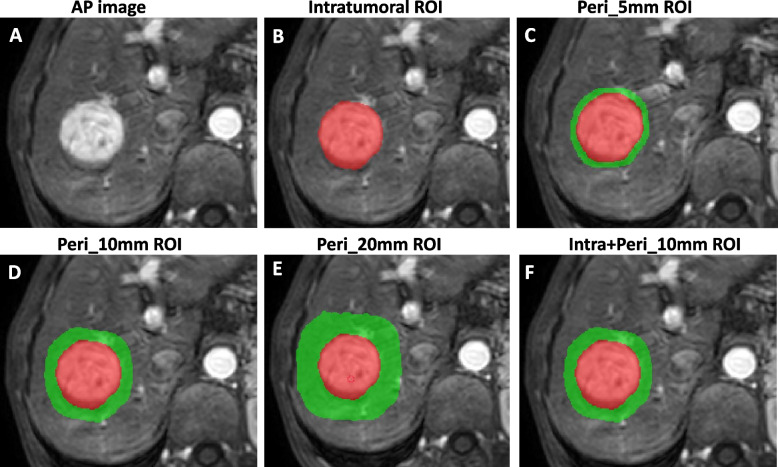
Examples of masks with different dilation distances on MR images. The red region represents the intratumoral region that was segmented by radiologists. The green-colored ring-like regions indicate the multiple peritumoral regions obtained with dilation
